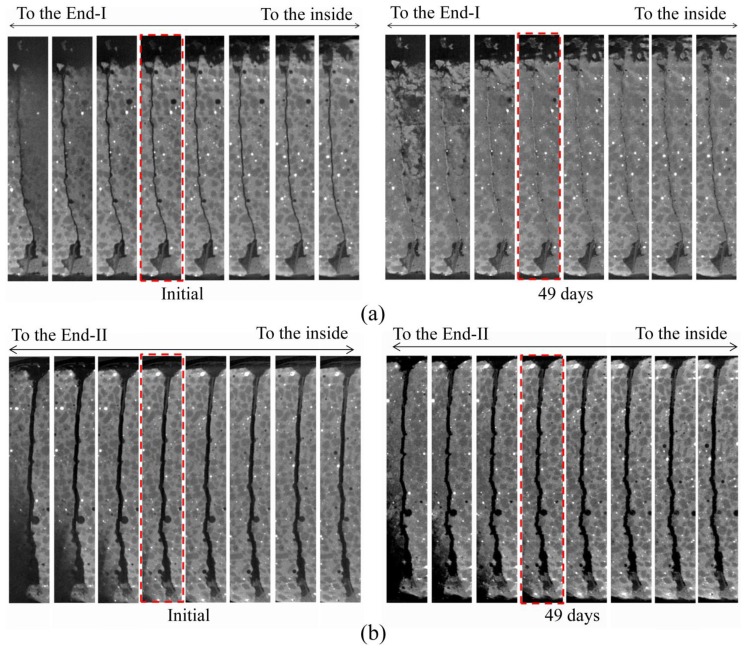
Comparison of CT images of macro-fracture at initial stage and after being kept in seawater for 49 days for (a) End-I and (b) End-II. Height and width of each image are 14 and 2 mm, respectively. Note that the red dashed lines correspond to the top cross sections of each region of interest (ROI) in Figure 5.
